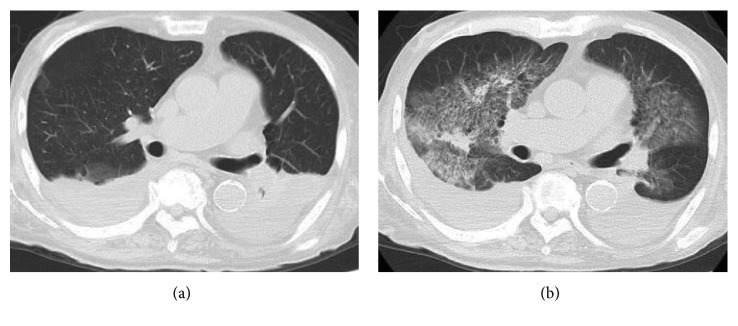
Chest CT at the time of admission (a) and 14th hospital day (b).
